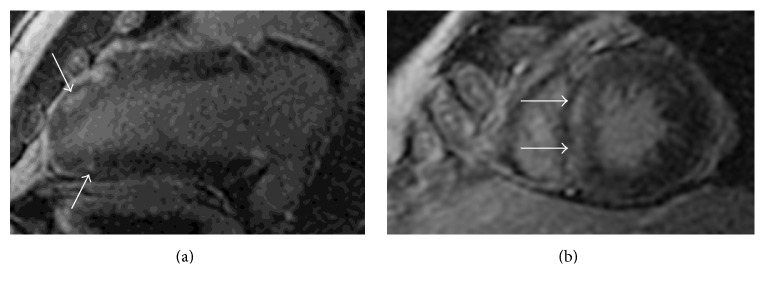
Cardiac magnetic resonance imaging with demonstration of patchy and some full-thickness late gadolinium enhancement of the (a) distal anteroapical wall segments, inferoapical segment, and (b) mid septum (arrows).
